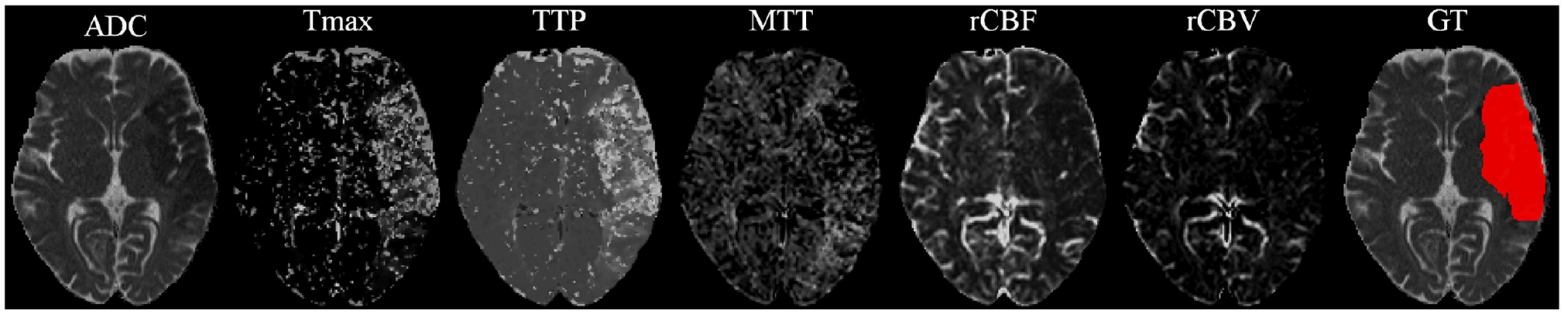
MRI parametric maps of a stroke patient with TICI score 3, and the respective manual segmentation.
